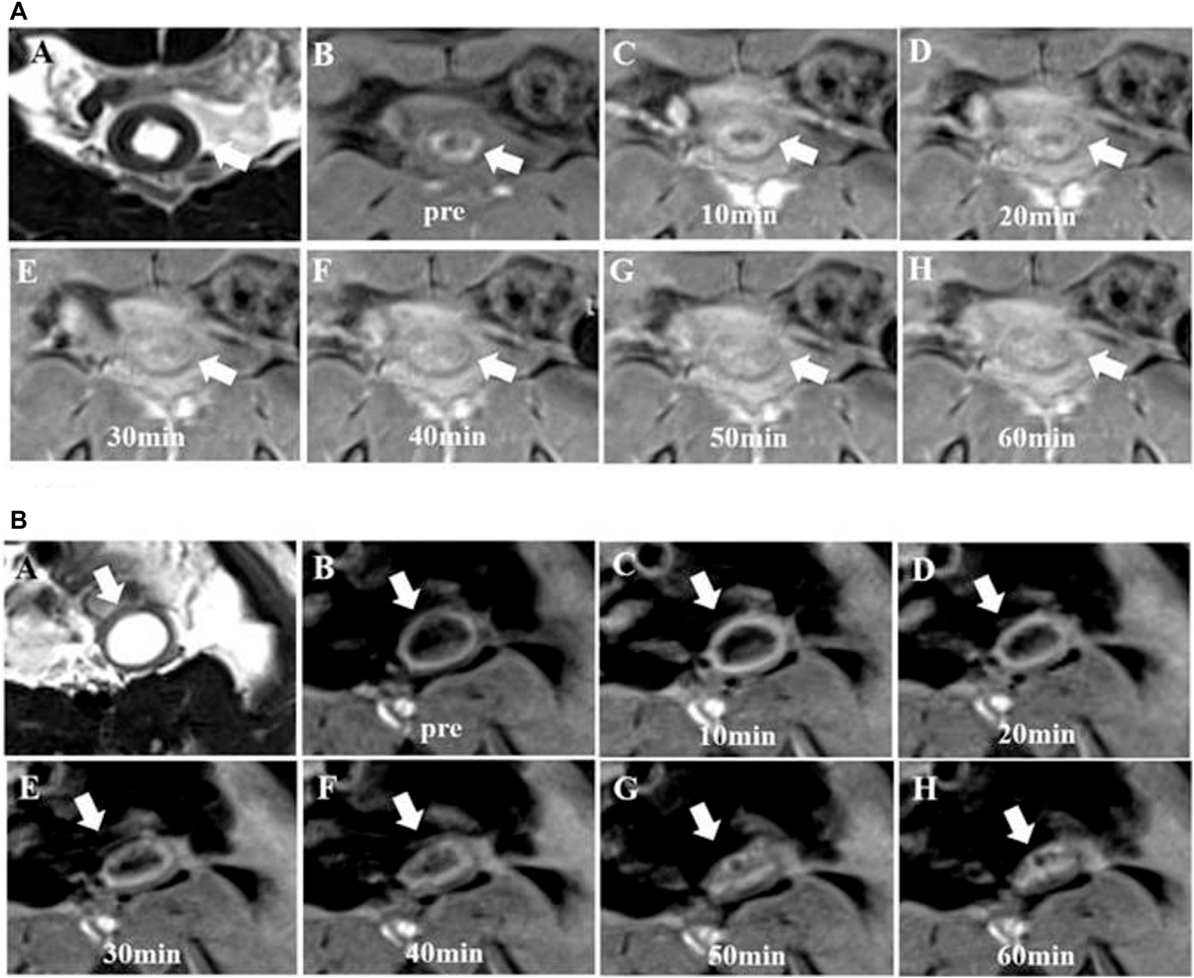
Signal intensity maps of the fibrotic bowel wall obtained from MR imaging. A to H are maps of moderate bowel wall fibrosis. (A), T2-weighted imaging enhanced from EP-3533 enhanced MR imaging: T2-weighted imaging (A) and T1-weighted imaging (B) showed thickening of the bowel wall. The mucosa of the bowel wall showed hyperintensity on T1-weighted imaging due to the mucus adhering to the bowel wall surface. After EP-3533 injection, the bowel wall fibrosis marked by the red arrows showed obvious enhancement (C–G). (B), T2-weight imaging from Gd-DTPA enhanced MR imaging: A to G are the maps of the other moderate bowel wall fibrosis pre- and post-injection of Gd-DTPA. After Gd-DTPA administration, the bowel wall fibrosis showed slight enhancement.
